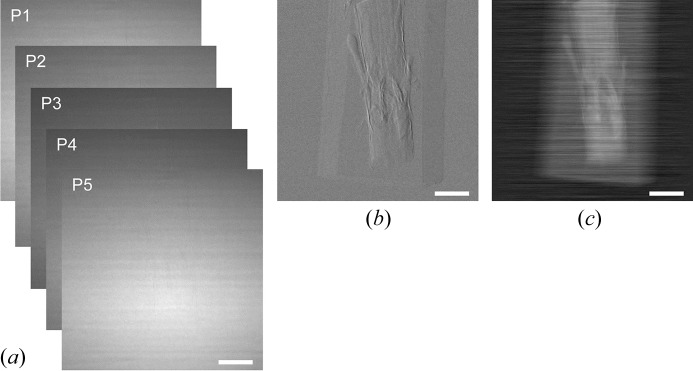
X-ray phase-contrast images of spinal cord after scaffold implantation. (*a*) Raw images of the spinal cord after scaffold implantation observed with the X-ray grating interferometer. (*b*) Differential phase-shift image obtained by a fringe-scan calculation. (*c*) Phase-shift image obtained by one-dimensional integration of (*b*). Scale bar = 2 mm.
